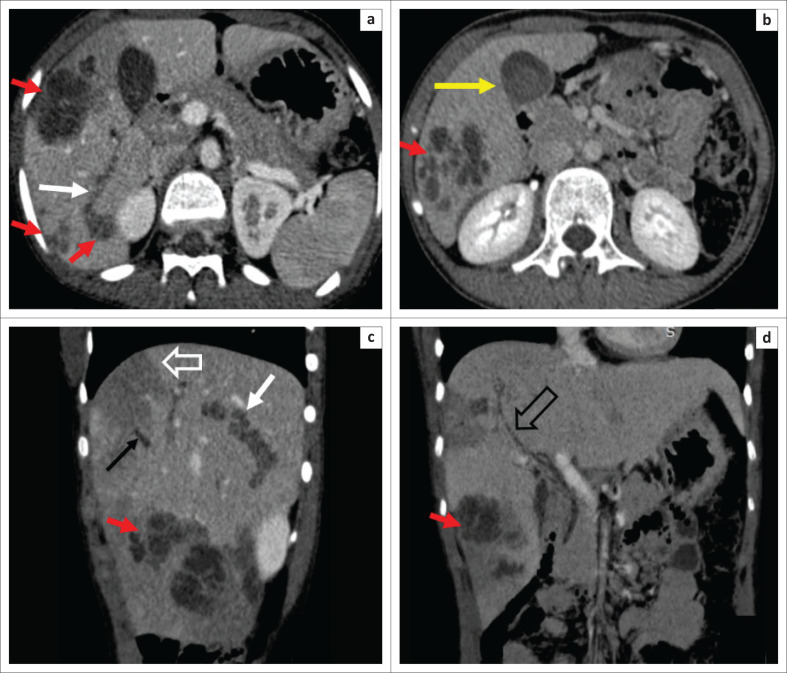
Axial (a, b) and sagittal (c) portal venous phase CT images showing multiple clustered hypodense lesions (red arrows) in the right lobe of the liver with indistinct margins. Few linear hypodense tracks (white arrows a, c) are also seen, consistent with the migration of larvae. There is hyperdense content within the gall bladder (GB) lumen (yellow arrow b) sludge or haemorrhage. A small non-enhancing portal vein (PV) segment is noted on sagittal image c in segment VIII, suggestive of segmental PV thrombosis (thin black arrow c). Associated transient hepatic arterial difference (THAD) is also found (open white arrow c). Coronal minimum intensity projection (MinIP) image (d) showing mildly dilated central biliary radicles (open black arrow).
